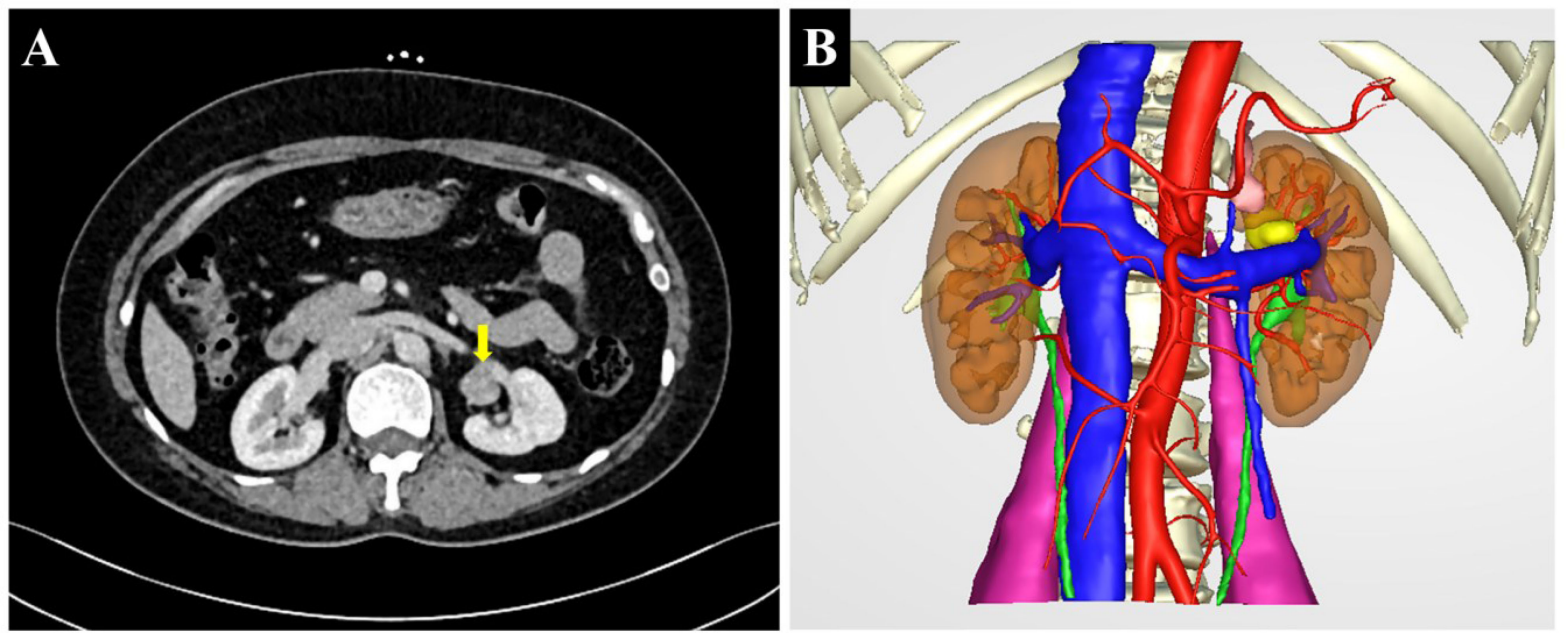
(A) Multislice spiral computed tomography (CT) showing a lobulated soft tissue density nodule above the hilum in the medial upper pole of the left kidney (arrow). (B) The preoperative three-dimensional reconstruction image showing the tumor in the renal sinus (yellow).
